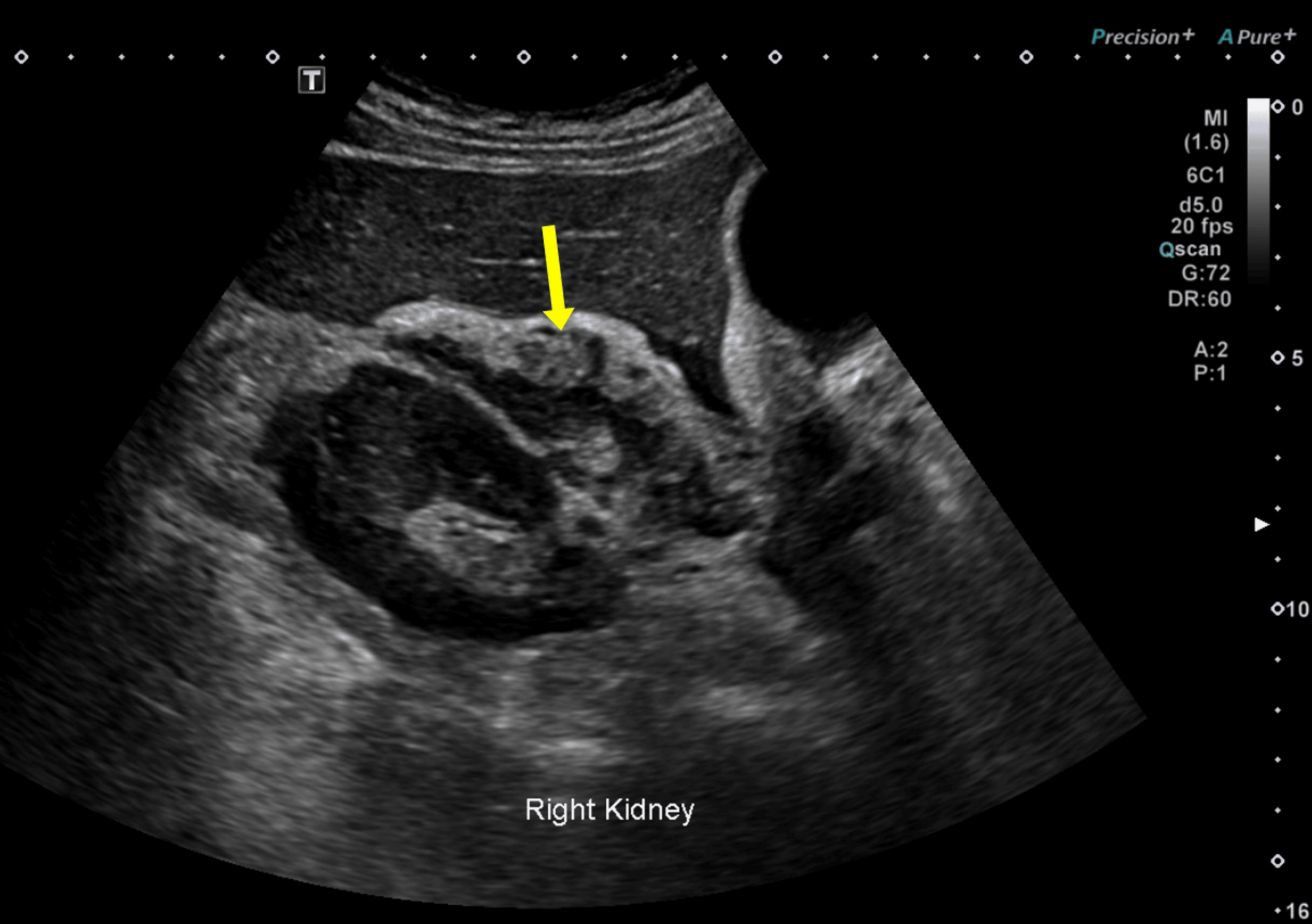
Follow-up ultrasound performed two weeks later demonstrating a heterogeneous suprarenal lesion (yellow arrow).
